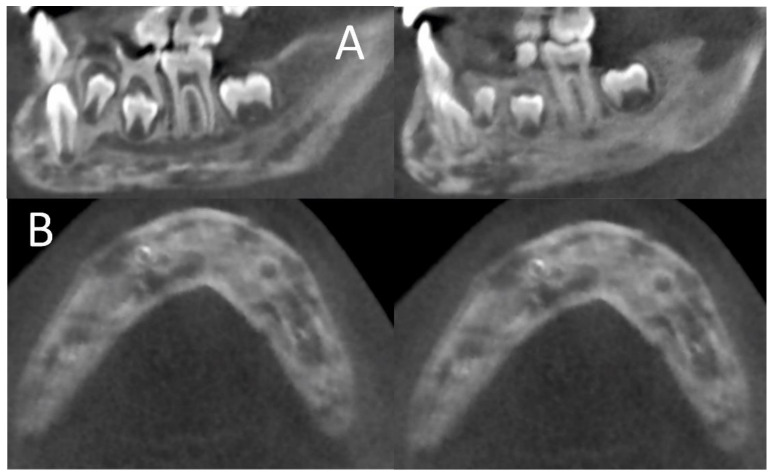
Initial Cone beam computed tomography (CBCT) examination shows osteolytic and sclerosis lesions (“onion skin”); A—sagittal sections through the body and ramus of the mandible; B—cross sections through the body of the mandible.
